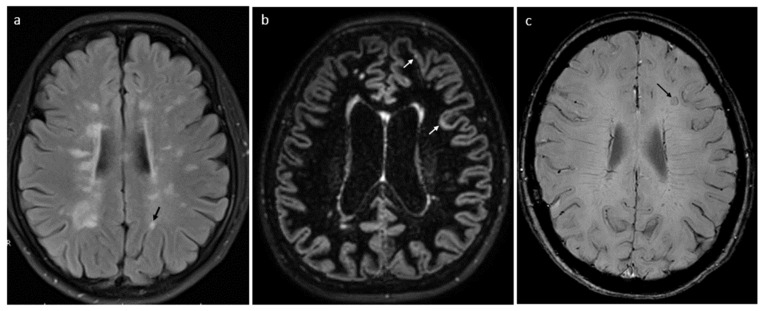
Patient with PPMS. MR images of the brain showing the following: (a) multiple demyelinating plaques in periventricular and juxtacortical (arrow) locations on FLAIR sequence, (b) cortical plaques (arrows) on DIR sequence and (c) a paramagnetic rim lesion (arrow) on SWI sequence; own resources.
